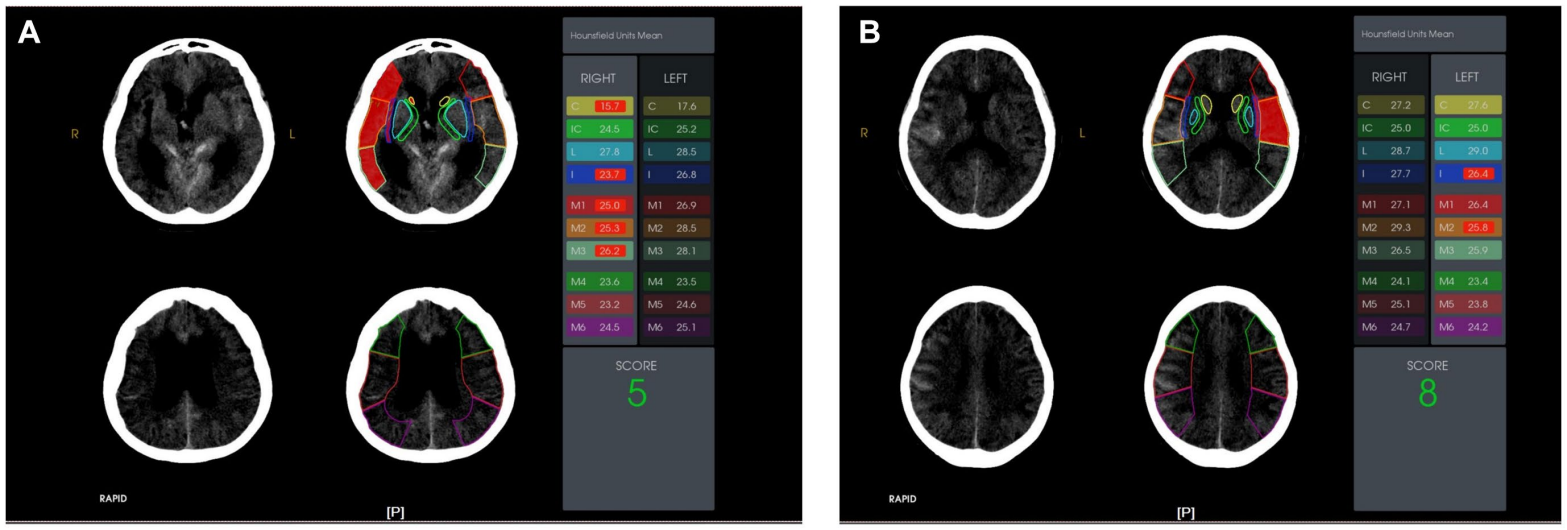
The ten Alberta Stroke Program Early Computer Tomography (ASPECT) score regions were outlined on the non-contrast computed tomography head images. The Hounsfield Unit (HU) value difference in the ten ASPECT score regions are shown. Case presentations: (A) A 62-year-old female with a right caudate HU value of 15.7 on admission. She suffered from stress-related gastrointestinal bleeding after an aneurysmal subarachnoid hemorrhage; (B) A 43-year-old male with a right caudate HU value of 27.2 on admission. He did not suffer from stress-related gastrointestinal bleeding after aneurysmal subarachnoid hemorrhage.
